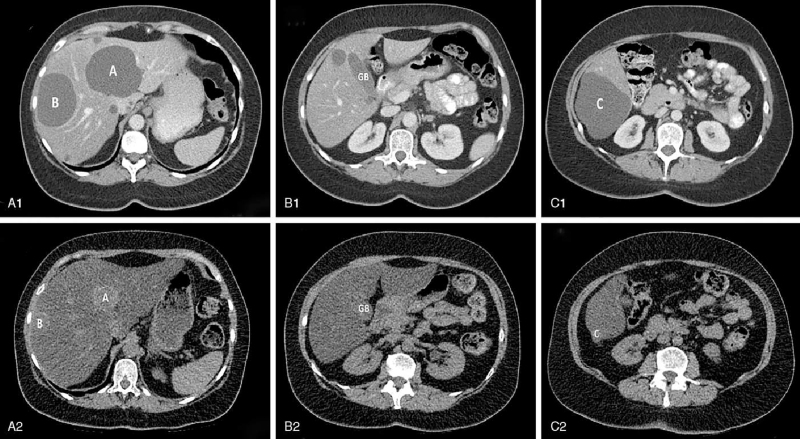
CT scans at start of tamoxifen, and 4.6 years later. 1A–C, Axial images of a contrast enhanced CT-scan of the liver (venous phase), performed just at the start of tamoxifen. Three large cysts in segment 4 (cyst B), 6 (cyst C), and 7 (cyst A) and multiple small cysts are present in the liver. The large, irregular cyst in segment 6 (cyst C) is either composed of multiple adjoining cysts or contains septations, caused by cyst bleeding. 2A–C, A low-dose CT-scan 4.6 years later. Due to pronounced hepatic steatosis, cysts appear relatively hyperintense. All cyst volumes were reduced, while only the cyst in segment 6 (cyst C) had been treated with drainage and sclerotherapy. The gallbladder (GB) has also been marked for reference on both scans.
